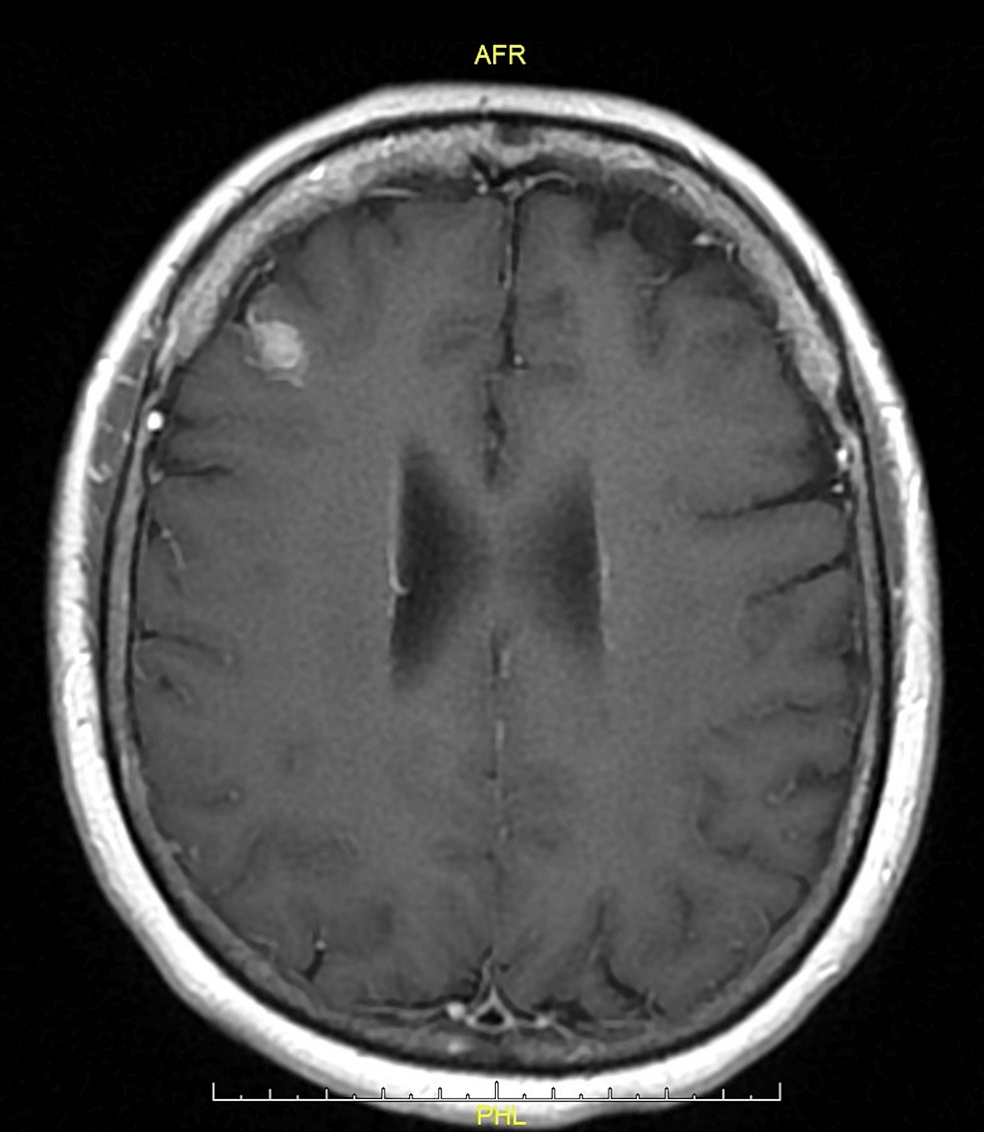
Magnetic resonance imaging T1 axial image of brain
